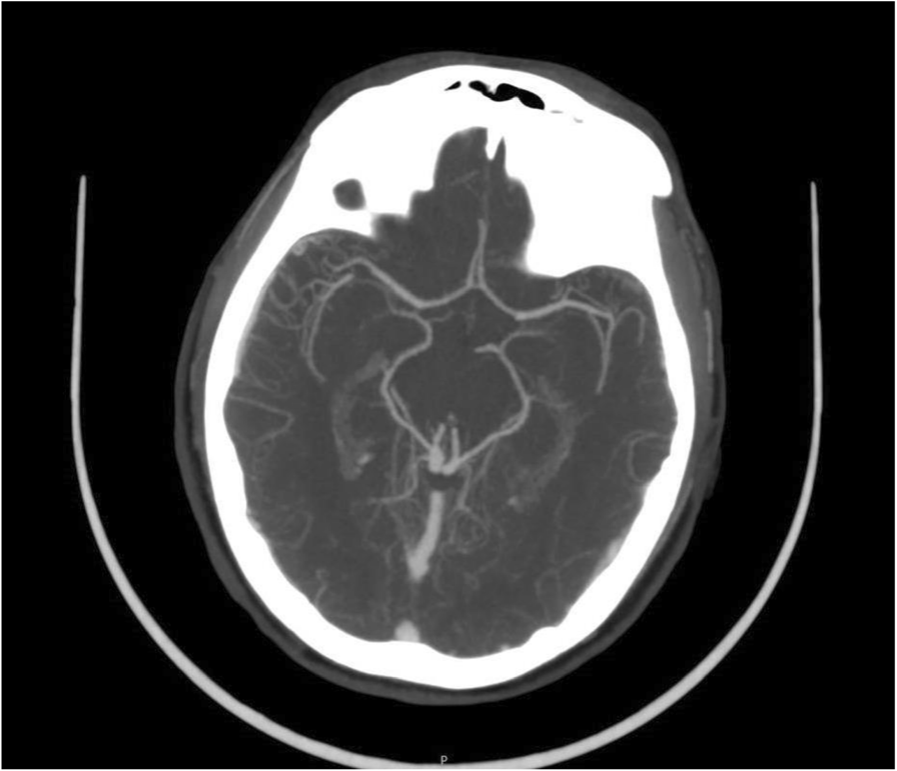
MRI angiography. Normal Willis polygon
